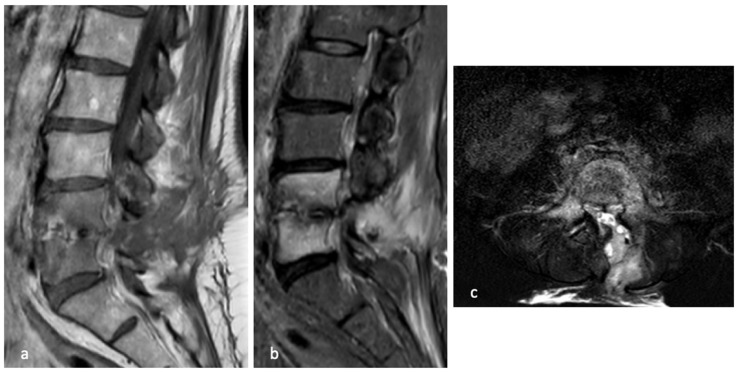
Post-operative MRI in which the surgical approach via and drainage of the intracanal abscess can be seen, in the sagittal T1 (a) and T2 (b) weighted sequences, as well as signal alteration of the vertebral bodies and disc.
